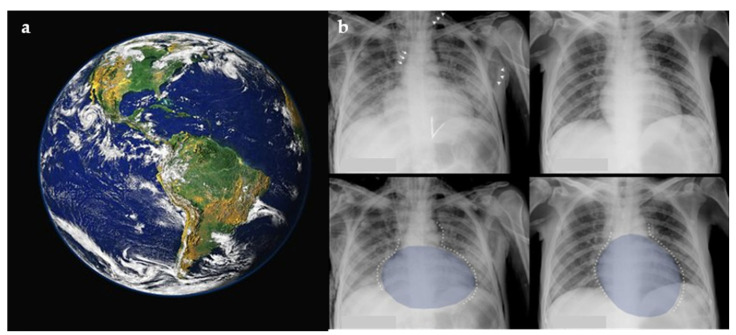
The Earth as it appears from outer space (a), with resemblance to the flattening and posterior displacement of the heart by the tension pneumomediastinum on a plain chest radiograph (b). Figure used with permission of the author G. A. O. Carillo; *The Lancet*, 2014 [19].
